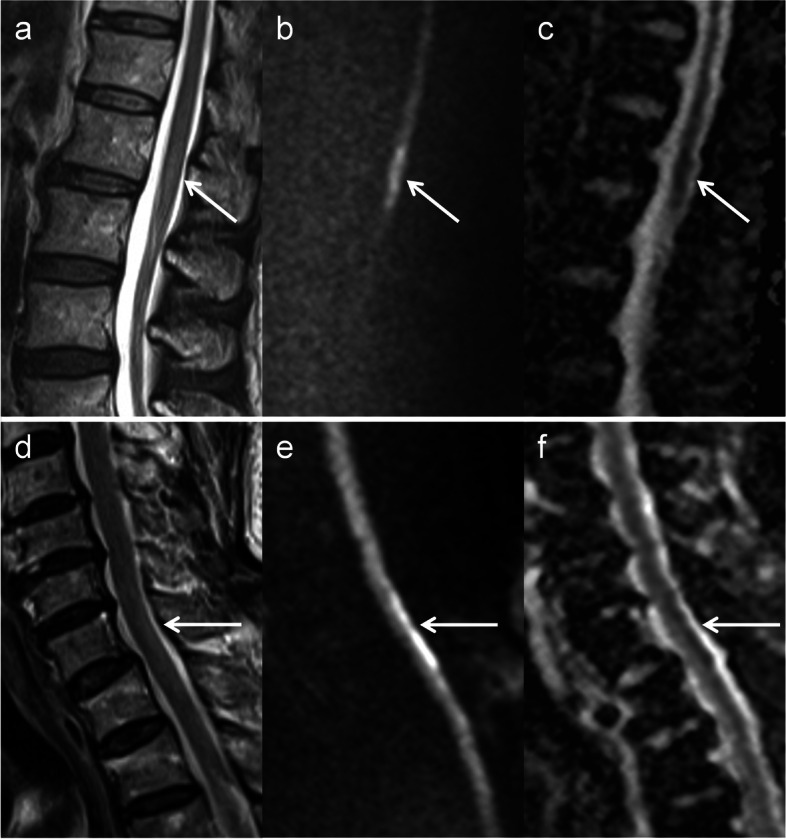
Early ischemic changes and posterior linear hyperintensity. MRI findings of a 73-year-old woman who noticed muscle weakness and paresthesia in the lower limbs were obtained 11 h from symptom onset (a–c). On T2-weighted images, the lesion was marginally high but not definitive (arrow, a); however, it was evident on DWI and ADC maps (arrows, b and c) and indicated spinal cord infarction. A 77-year-old man suddenly experienced back pain and gait difficulty and underwent MRI 4 days after symptom onset. Slight T2 hyperintensity was observed at the C7–T1 level of the cord (arrow, d), which was clearly high on DWI (arrow, e; posterior linear hyperintensity) and low on ADC maps (arrow, f). The lesion suggested ischemia of the posterior spinal artery. ADC, apparent diffusion coefficient; DWI, diffusion-weighted imaging; MRI, magnetic resonance imaging
